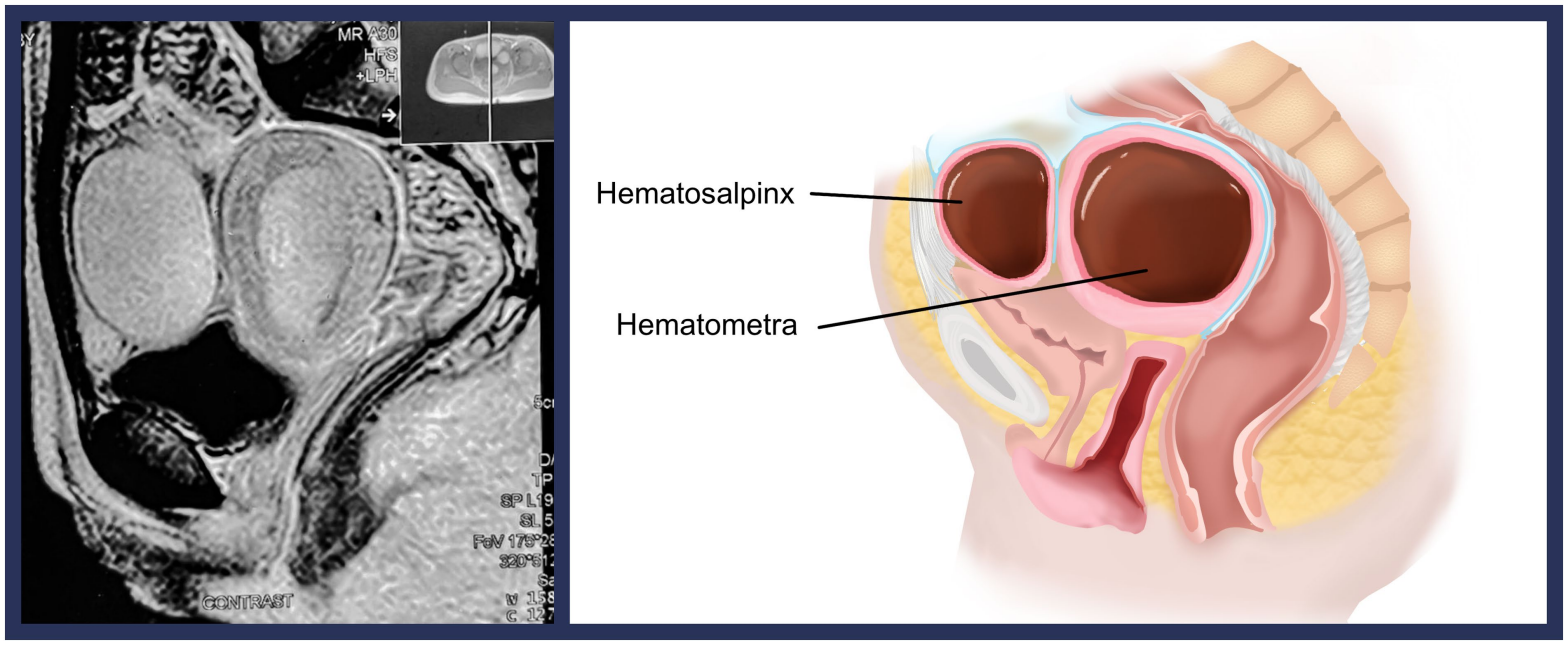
Magnetic resonance imaging demonstrating the hypertrophic uterus with the hematometra and the left hematosalpinx (left). Additionally, a visual demonstration of the malformation is presented (right).
